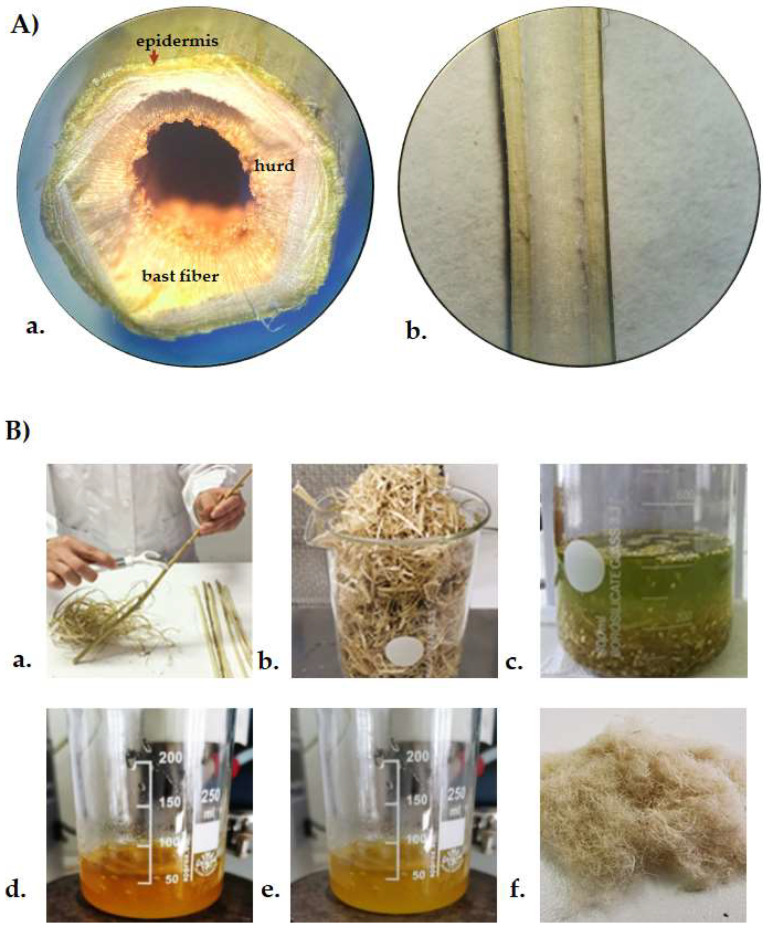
(A) Hemp Stem (a) transversal section; (b) longitudinal section; (B) Hemp cellulose extraction steps. (a) Hemp stem peeling; (b) Ultrasound-assisted maceration; (c) Alkaline treatment; (d) Acid hydrolysis; (e) Hemp cellulose recovery; (f) Representative cellulose sample.
